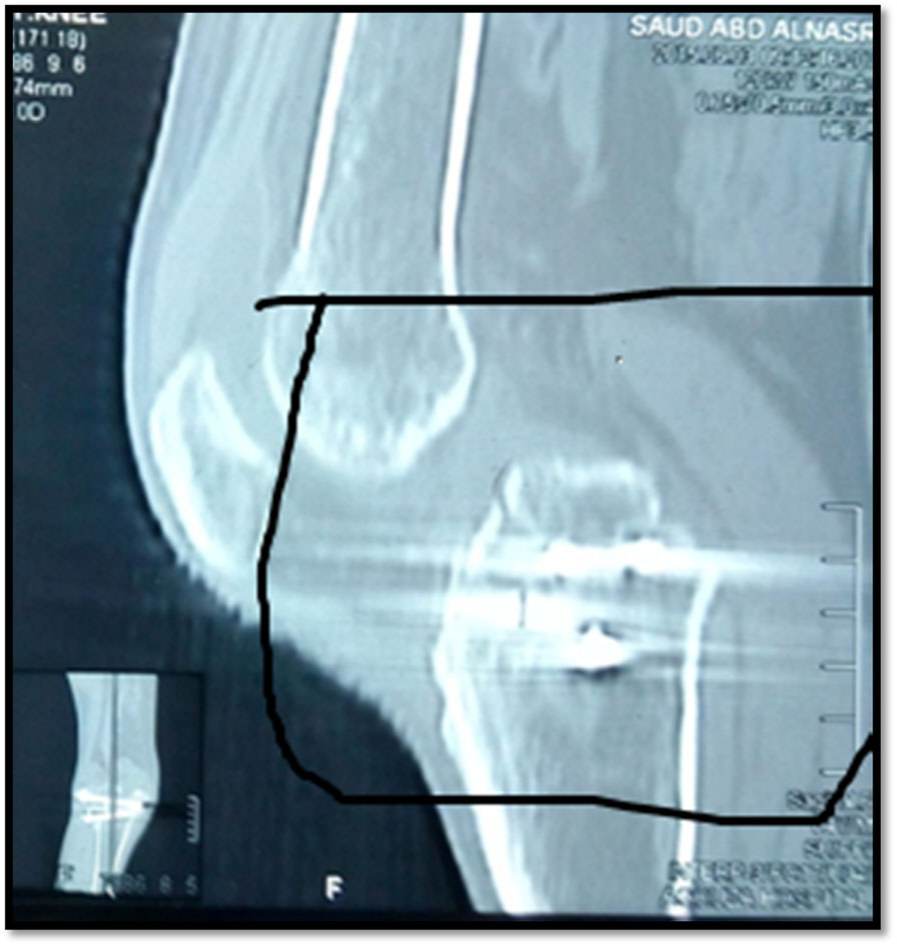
CT scan of posttraumatic unstable knee
